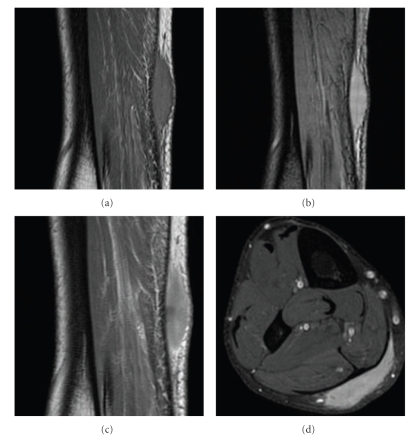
Sagittal T1 (a), T2* (b) weighted images depict a fusiform mass over the Achilles tendon. Postcontrast sagittal T1 (c) and axial fat-saturated T1-weighted images demonstrate a moderate and homogeneous enhancement.
